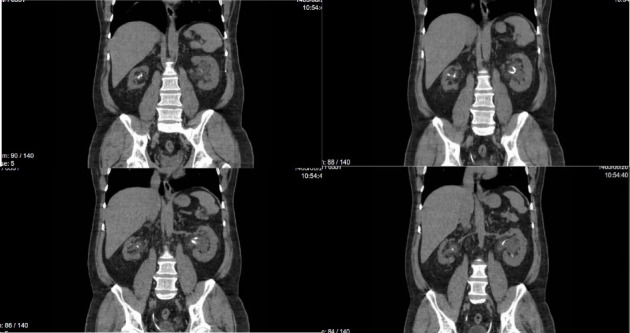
Noncontrast CT scan of abdominal pelvic showed a patent urinary tract with no evidence of encrustation or knotting in left kidney.
